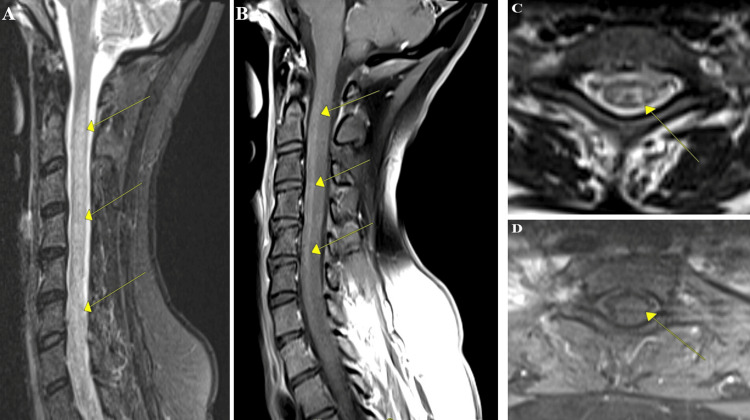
MRI of the cervical spine with and without contrast. A-B) Diffuse and extensive T2 hyperintensities in the cervical and upper thoracic spine with mild contrast enhancement as demonstrated by the yellow arrows on sagittal cuts appreciated; C-D) axial MRI images at C6 with T2 hyperintensities with mild contrast enhancement shown with the yellow arrows.
